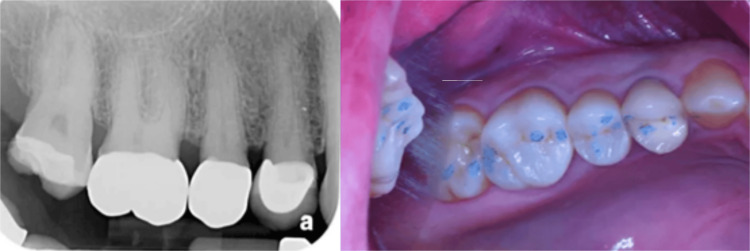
Radiograph and clinical picture of previously restored teeth in the upper right quadrant
